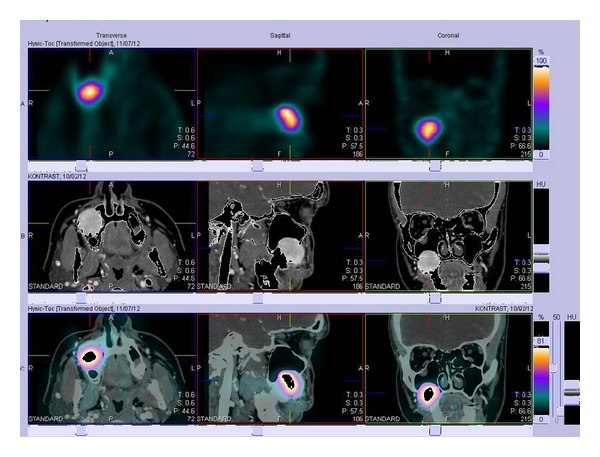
Fusion of CT and SRS scans: accumulation of radiotracer in the tumor.
